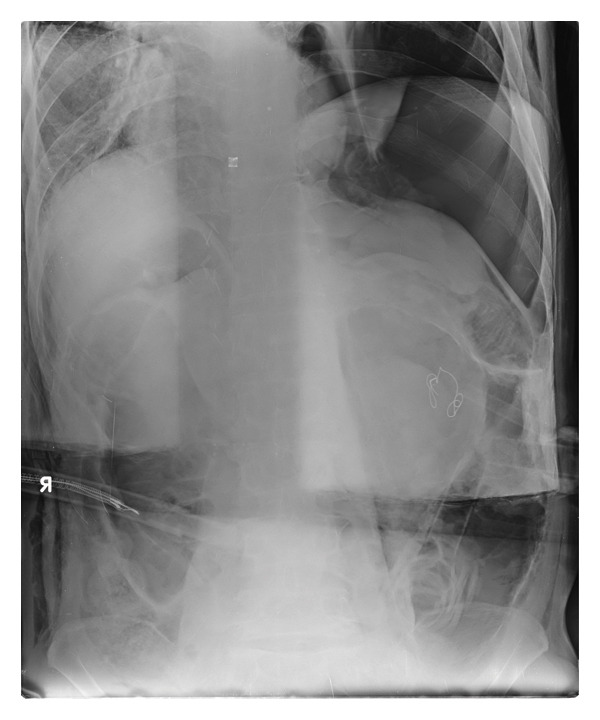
Portable abdominal radiograph obtained at the conclusion of surgery demonstrating a marked rightward mediastinal shift consistent with tension pneumothorax. Displacement of the heart and mediastinal structures suggests elevated intrathoracic pressure. The image was acquired in the prone position and horizontally inverted to maintain anatomical orientation.
